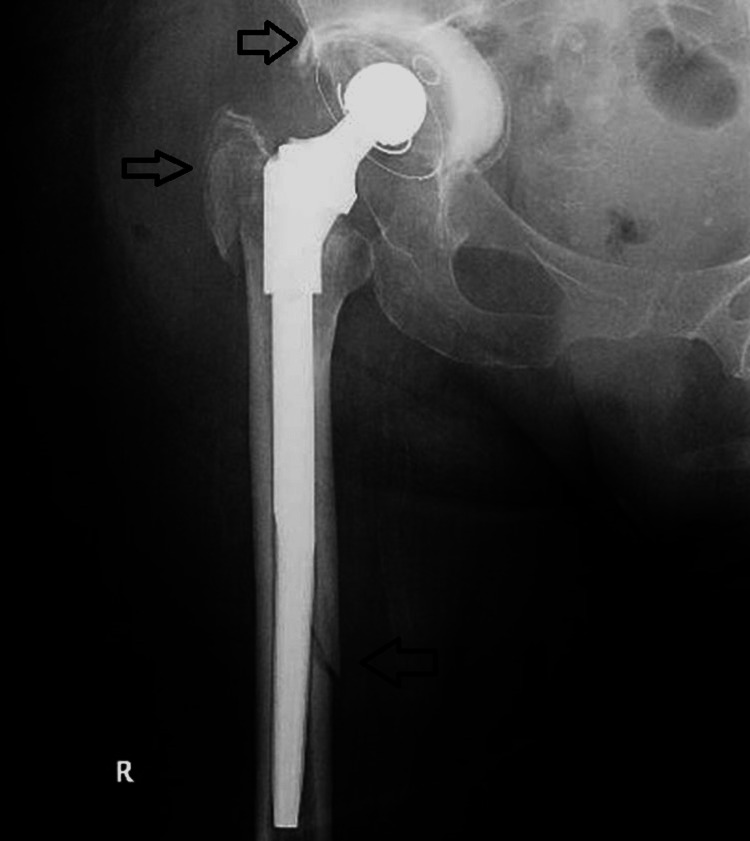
Postoperative right hip X-ray.
